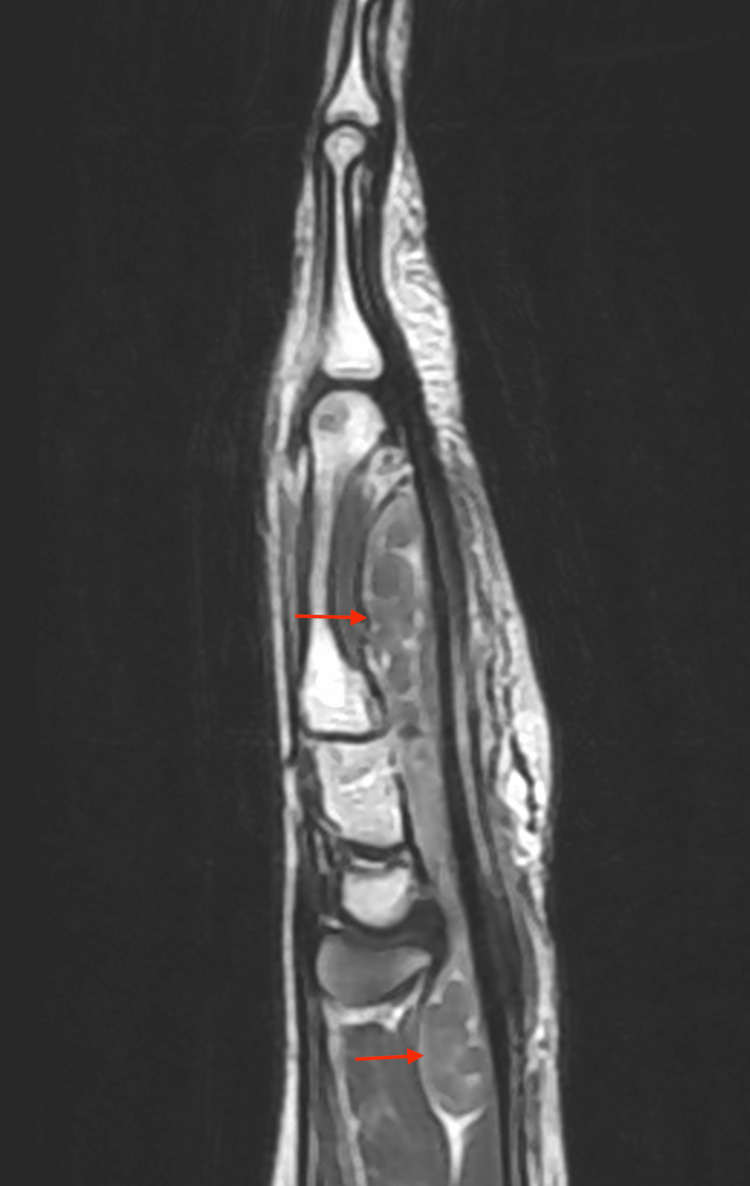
MRI of the left hand in sagittal view showing diffuse thickening and non-enhancing nodules in the tendon sheath of the flexor digitorum profundus and flexor digitorum superficialis
